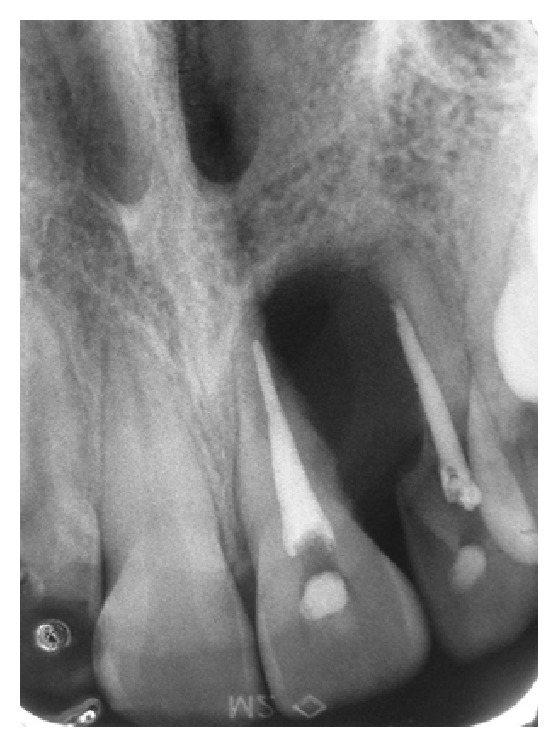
Periapical radiograph showing radiopaque image of the roots of teeth 21 and 22, which are compatible with root canal filling material, obtained from a radiolucent image of the blurred boundaries in the region of the upper incisors.
